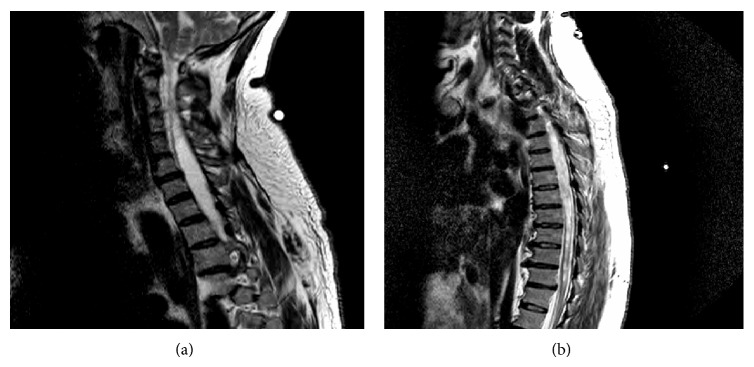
(a) MRI of the cervical spine showing a 9 mm inferior herniation of the cerebellar tonsils through the foramen magnum (Type I Chiari Malformation), with extensive cervical and superior thoracic spinal cord syringohydromyelia. (b) Thoracic spine MRI revealing extensive syringohydromyelia involving the entire thoracic spinal cord. The syringohydromyelia is more prominent in the superior midportion of thoracic spinal cord. T11-T12 central disk protrusion with thecal sac compression and slight ventral spinal cord compression.
